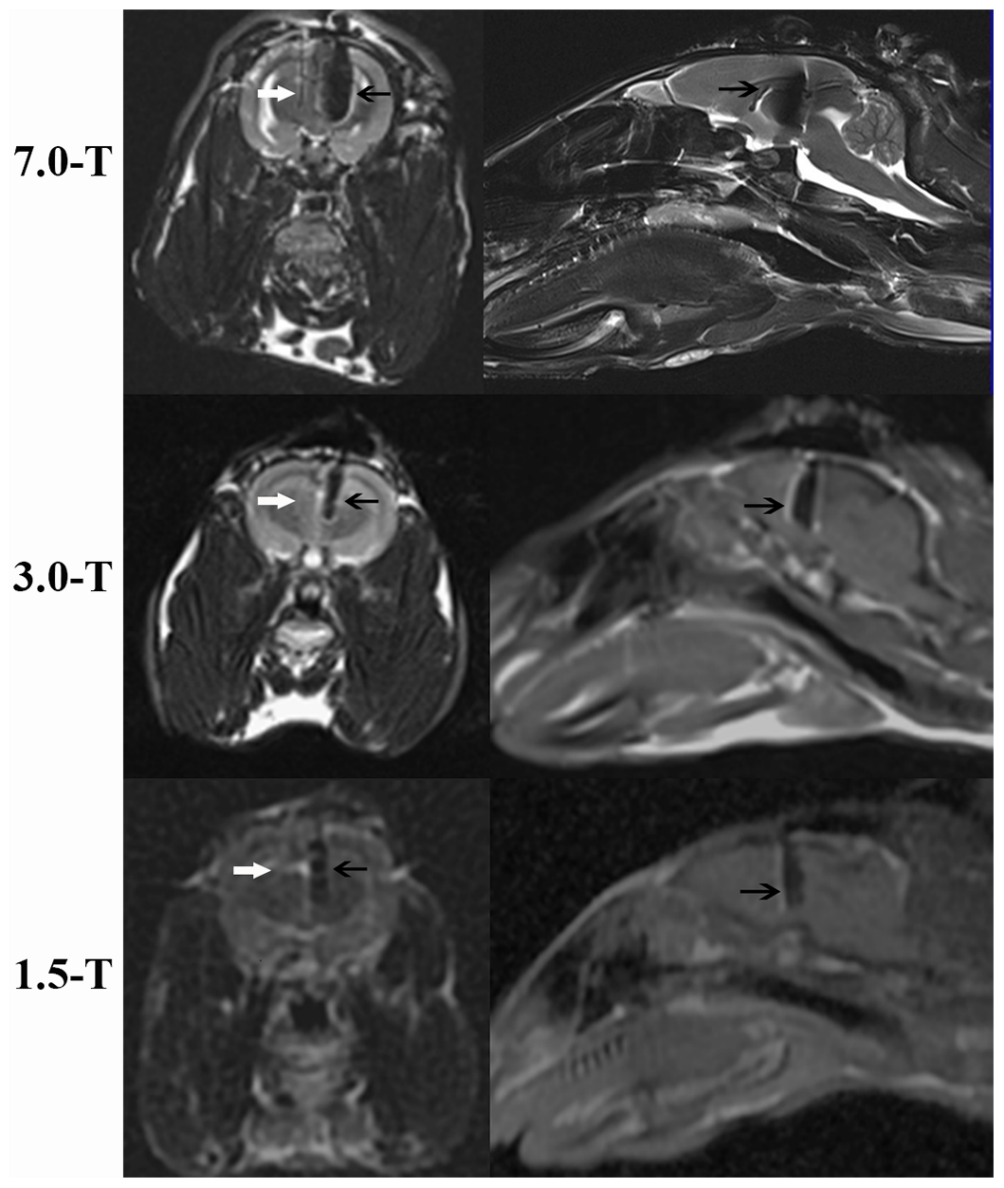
MRI (sagittal and axial sections) at levels of 7.0T, 3.0T and 1.5T. This image demonstrates the position of the lead (leftward, black arrow) that was covered by the artifact of the lead and the puncture passage (rightward, white arrow), which was unclear in the 3.0T and 1.5T images.
